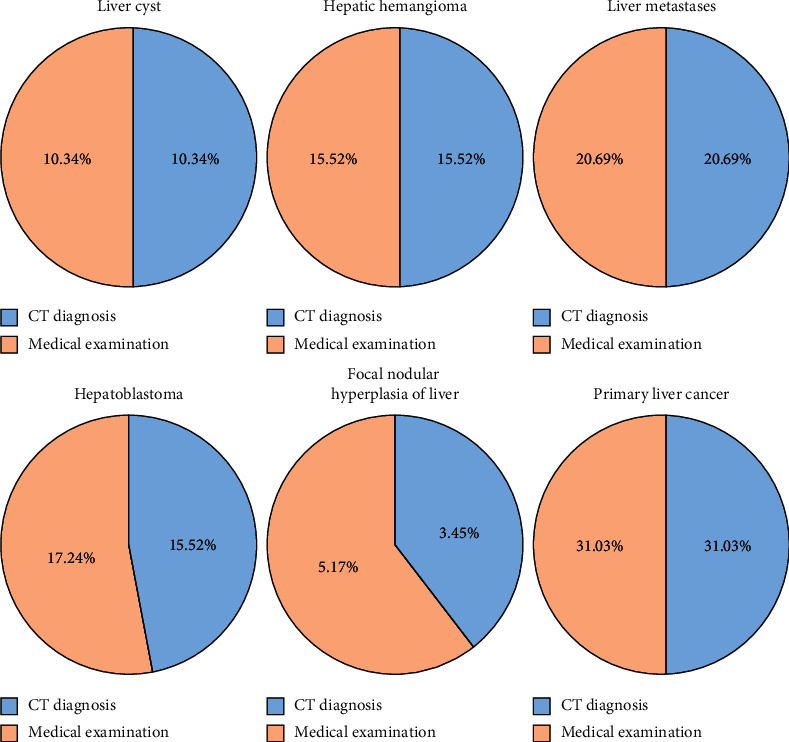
Diagnostic coincidence rate between CT scan and pathological results.
